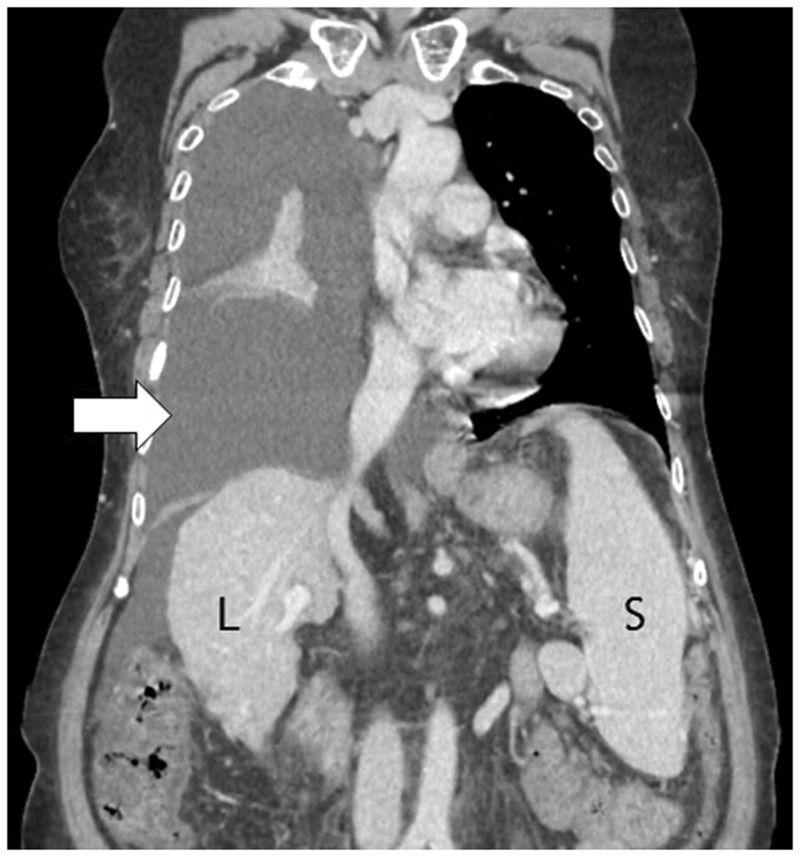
Hepatic hydrothorax. Coronal reformatted contrast-enhanced CT scan in a woman with liver cirrhosis showing gross right-sided pleural effusion (arrow). Also noted is presence of ascites, cirrhotic changes in liver (L) and splenomegaly (S).
